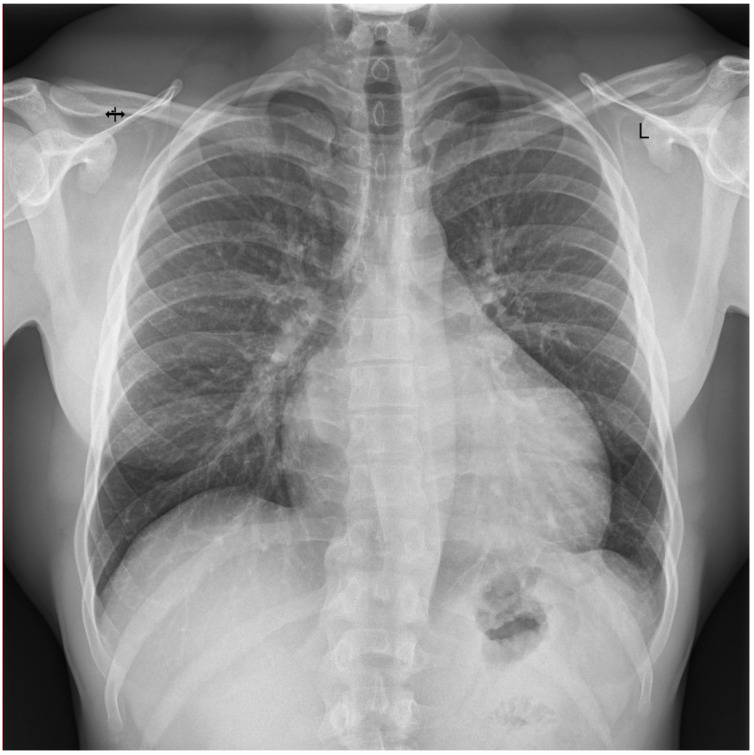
Chest X-ray of Patient 2: anteroposterior view. Kerley B lines are visible.
